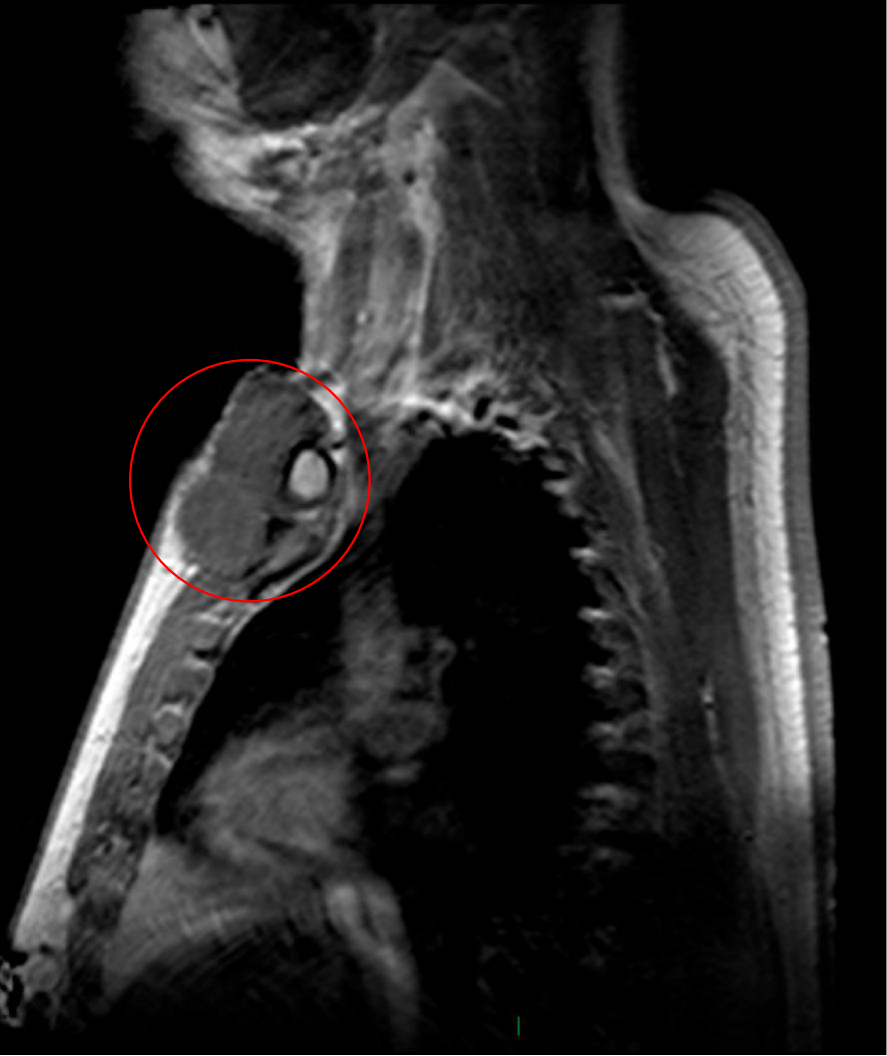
Magnetic resonance imaging showing the presence of locally advanced tumor growth with a close relation to the clavicula and internal jugular vein.
